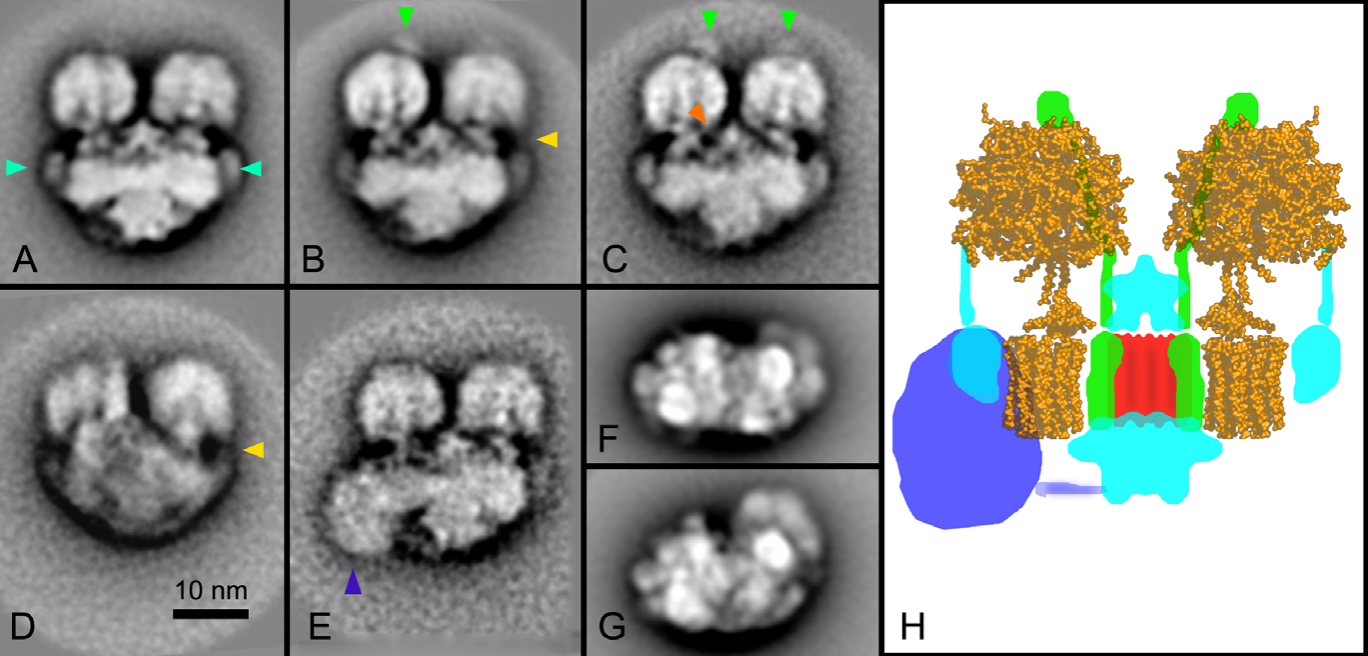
2-D projection maps of dimeric ATP synthase from *Tetrahymena thermophila*. (A–E) represent the side view, (F) top view, and (G) intermediate view. The Complexes were extracted either with digitonin (F, G), dodecyl maltoside (A, D, E), or the mixed dataset was used for image analysis (B, C). (E) Dimeric ATP synthase with an additional density next to the c subunit rotor of the left monomer (sum of 64 projections). (H) Interpretation of the projection (B, average of 3,254 projections) with the help of X-ray structure of yeast ATP synthase (PDB accession number 1QO1, [34]). Blue arrowheads (A) mark additional subunits on the extreme left and right positions of the c subunit rotors; green arrowheads (B and C), OSCP subunits; yellow arrowheads (B and D) point to an apparent connection between the domains seen at the extremities of the c rotor (cf., blue arrowheads in (A) and the F_1_ headpiece); the orange arrowhead (C), to a connection between the F_1_ part and the matrix exposed domain; and the dark blue arrowhead (E) points to an unknown large extra mass attached to F_o_. The bar represents 10 nm and applies to all frames.
